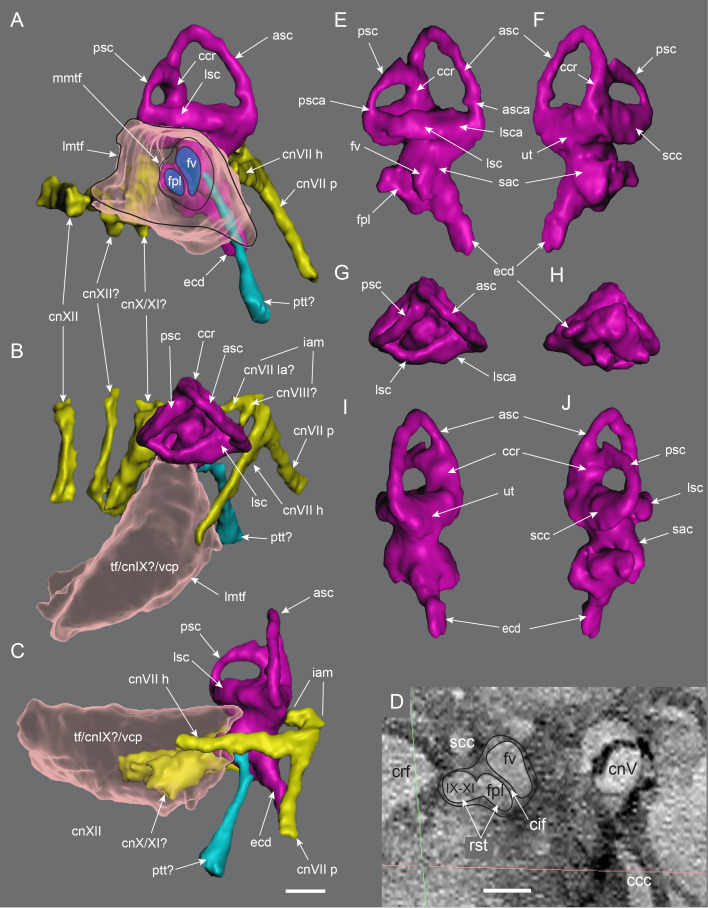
Volume rendered model and sagittal plane radiograph of the *Muttaburrasaurus langdoni* (QMF6140) right endosseous labyrinth. (A–C) Volume rendered model of tympanic fossa, endosseous labyrinth and myelencephalic cranial nerves in (A) lateral, (B) dorsal and (C) anterior views. (D) Sagittal plane radiograph in region of the *fenestra vestibuli*, *fenestra pseudorotunda* (*perilymphatica*) and *apertura anterialis* oblique to the long axis of and medial to the tympanic fossa. (E–J) Volume rendered model of endosseous labyrinth in (E) lateral, (F) posterior, (G) dorsal, (H) ventral (I) anterior and (J) posterior views. Abbreviations: amp, ampulla; asc, anterior semicircular canal; asca, region of ampulla of the anterior semicircular canal; ccr, common crus; ccc, cerebral carotid canal; cif, *crista interfenestralis*; crf, cranial fossa; ecd, endosseous cochlear duct; fpl, *fenestra perilymphaticum*; fv, *fenestra vestibuli*; iam, internal auditory meatus; lmtf, lateral margin of tympanic fossa; lsc, lateral semicircular canal; lsca, region of ampulla of the lateral semicircular canal; mmtf, medial margin of tympanic fossa; n#, cranial nerve and number (h = hyomandibular, p = palatine); ncf, neurocranial fossa; psc, posterior semicircular canal; psca, region of the ampulla of the posterior semicircular canal; ptt, pharyngotympanic tube; rst, *recessus scalae tympani*; sac, region of saccule; scc, secondary common crus; tf, tympanic fossa; ut, region of utricle; vcp, posterior cephalic vein. Scale bar equals one cm. MorphoSource DOI: 10.17602/M2/M788215.
